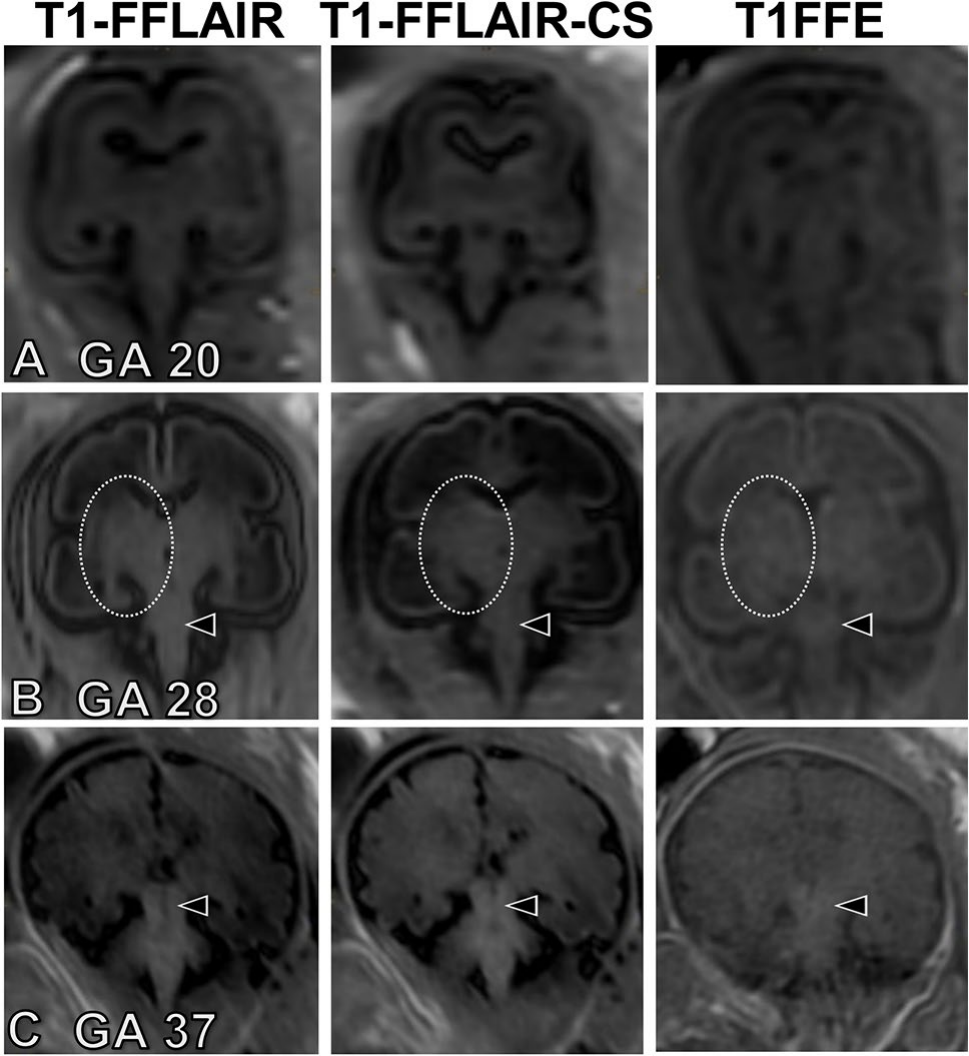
Coronal T1-weighted fast fluid-attenuated inversion-recovery (T1-FFLAIR), T1-weighted fast fluid-attenuated inversion-recovery with compressed sensing (T1-FFLAIR-CS), and T1-fast field echo sequence (T1-FFE) images at gestational age (GA) 20 (A), 28 (B), and 37 (C) showing the increase in signal intensity at the level of the brainstem and thalamus during fetal development, indicating progressive myelination. Note that the signal intensity of the brainstem (*arrowheads*) and thalamus (*circles*) is higher on T1-FFLAIR and T1-FFLAIR-CS compared to T1-FFE
